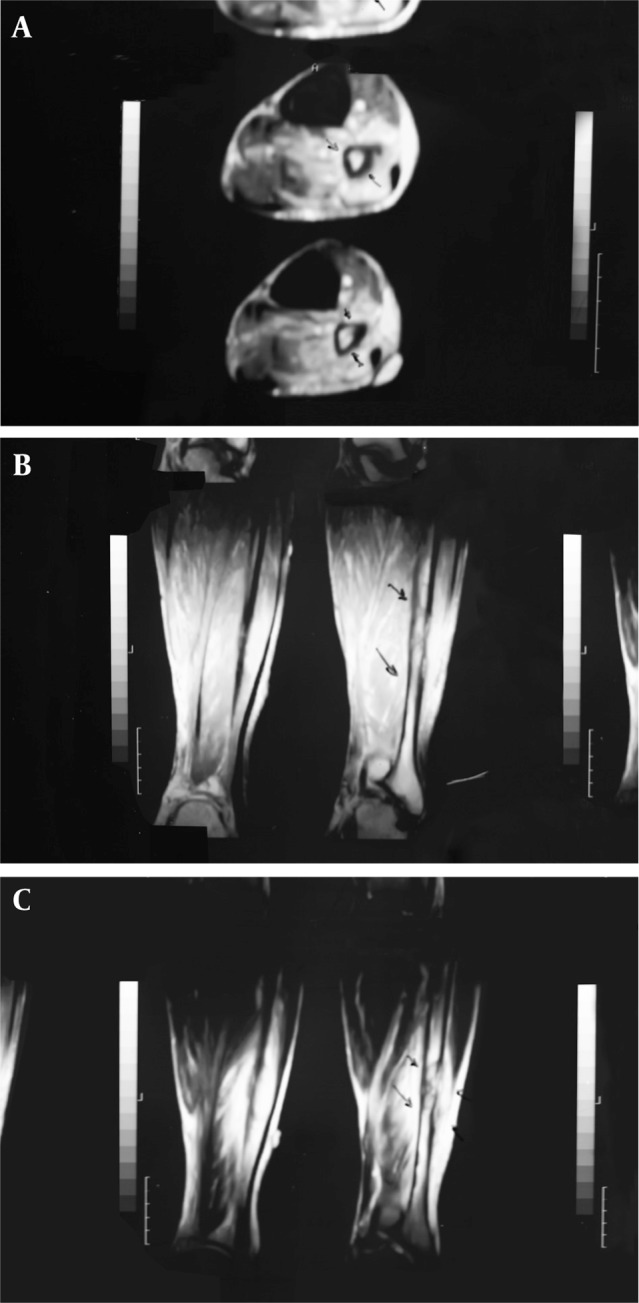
MRI of the right tibia A. Axial view, B. Sagittal view, C. Coronal view
